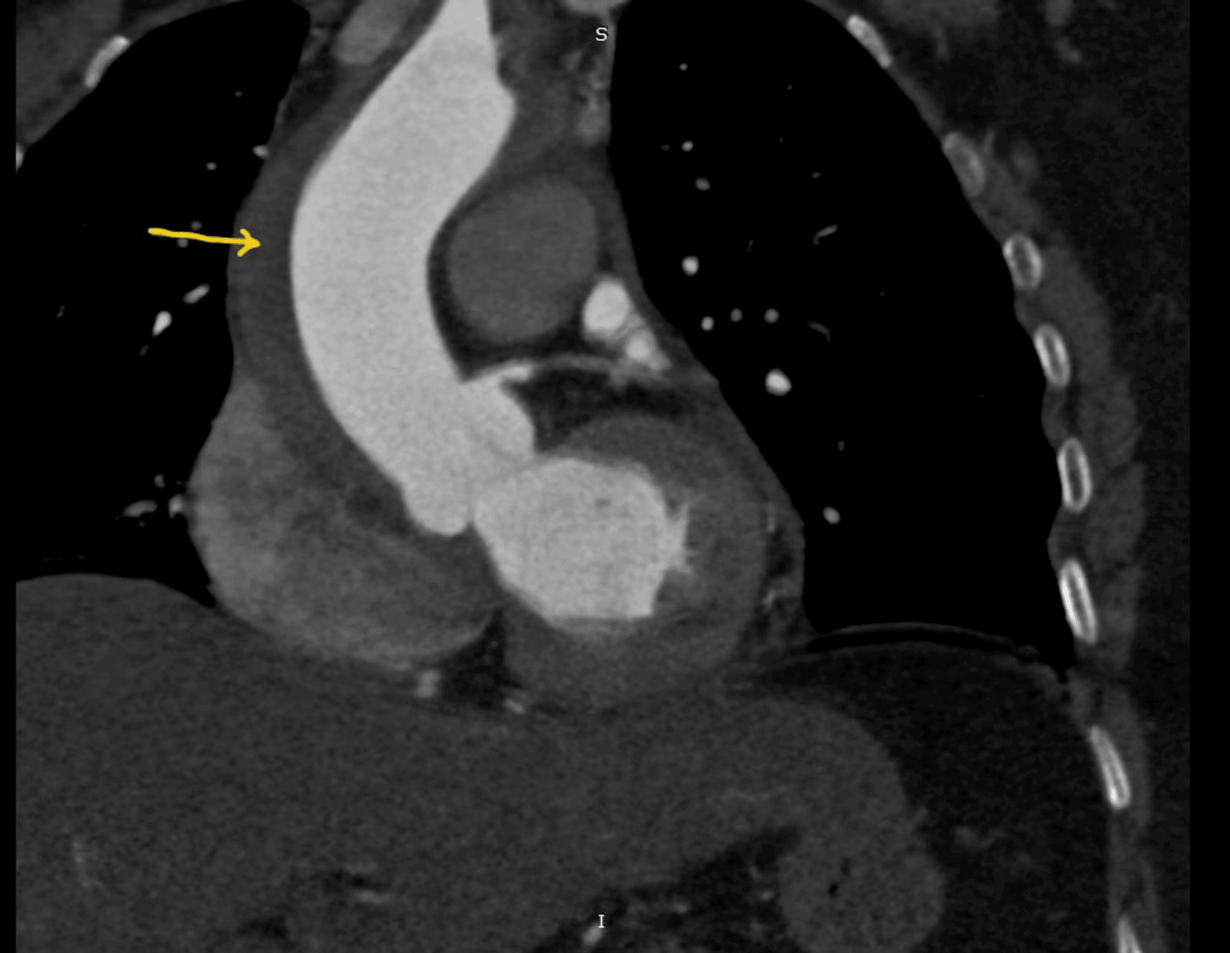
CT aortogram (coronal section) at the one-week follow-up showing the stabilized and significantly resolved dissection (arrow) in ascending aorta with no further extension or complications.
